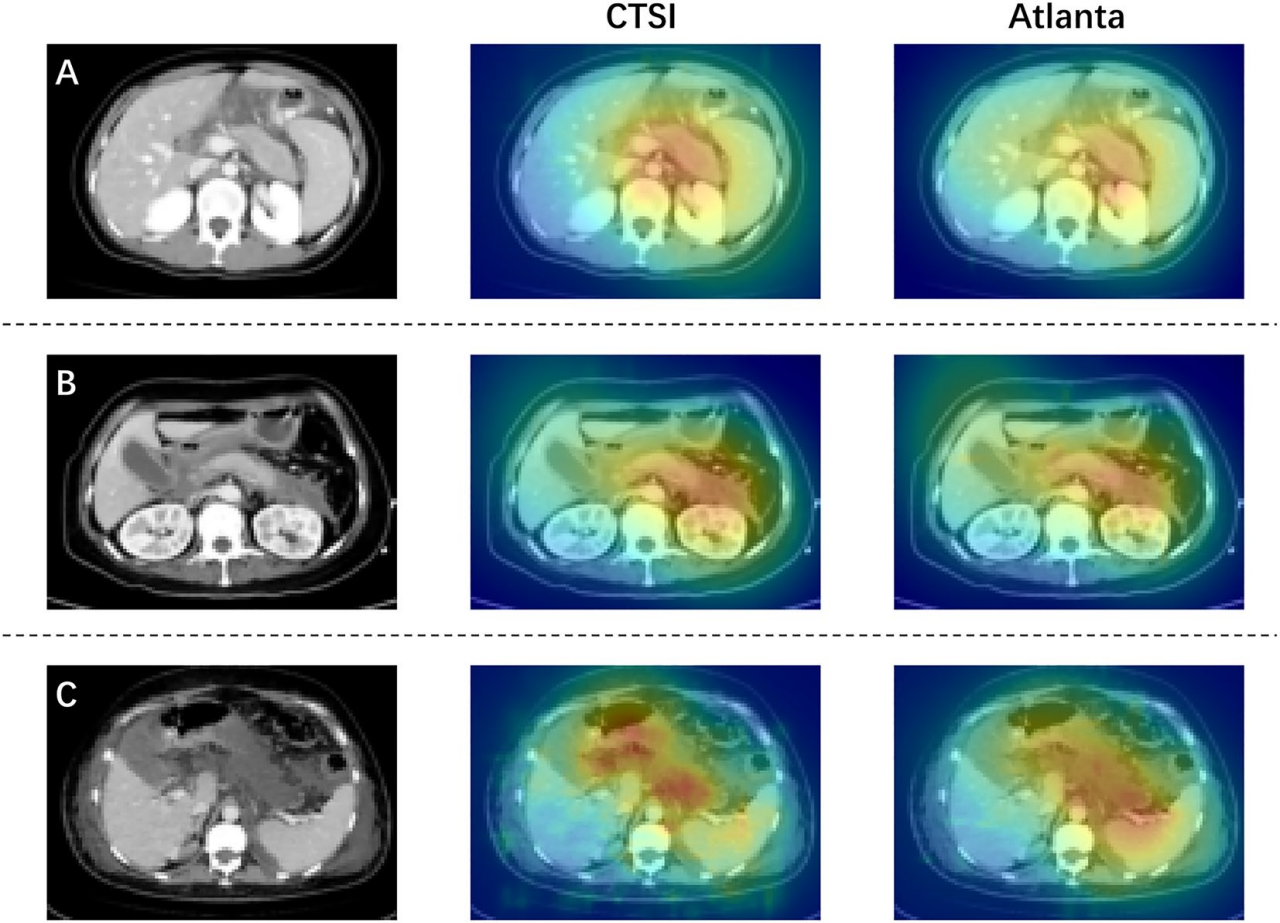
Visual explanations of model predictions using Grad-CAMs. The left column shows original CT scan images, the middle column displays visual explanations obtained from the model trained with CTSI-labeled CT scans, and the right column shows visual explanations from the model trained with Atlanta-labeled CT scans. (A) CT scan slice with mild pancreatic enlargement; (B) CT scan slice with peripancreatic accumulation; (C) CT scan slice with extensive pancreatic necrosis and peripancreatic accumulation.
